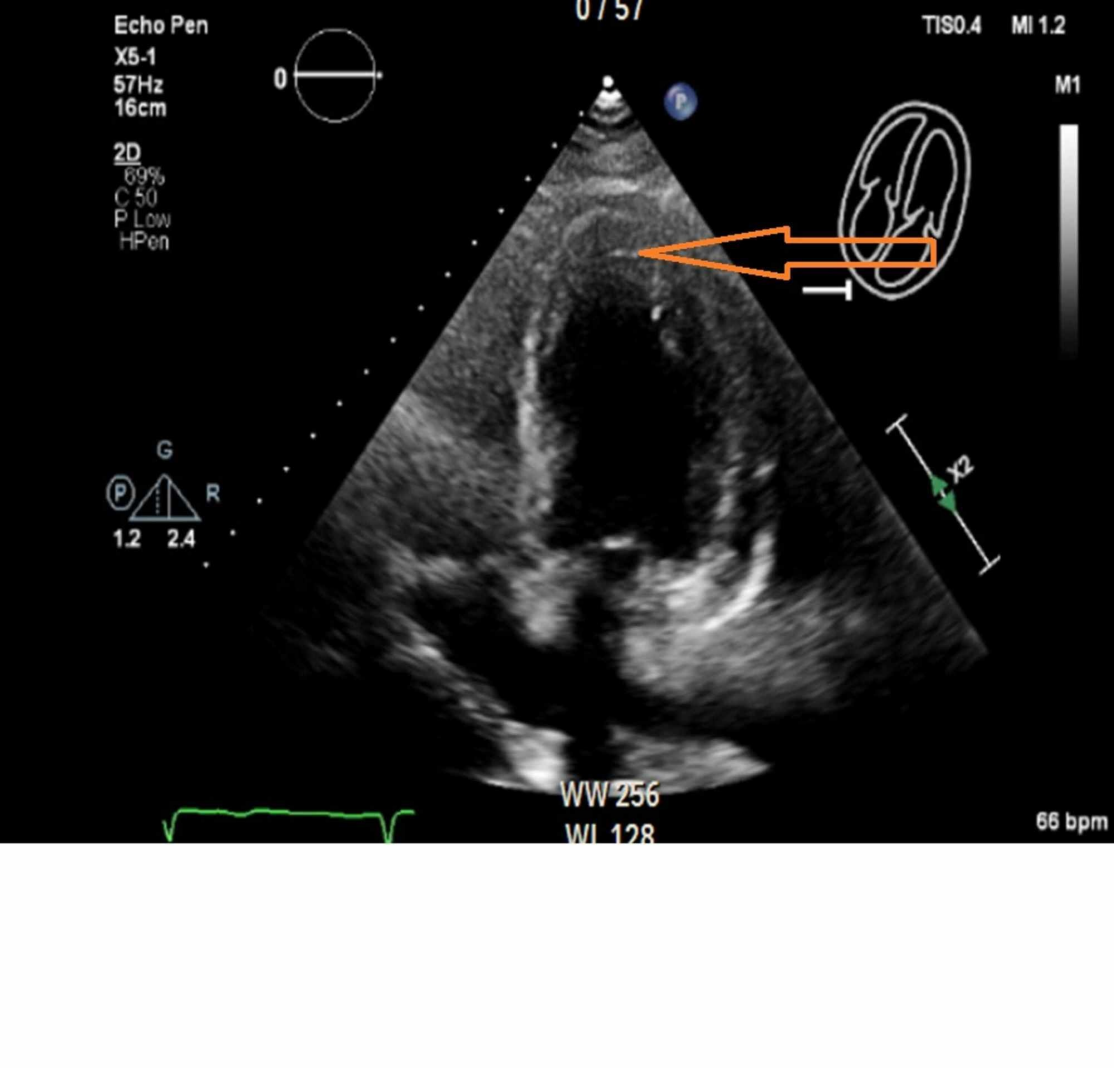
Echocardiogram The orange arrow showing akinesis of apical myocardial segment.
